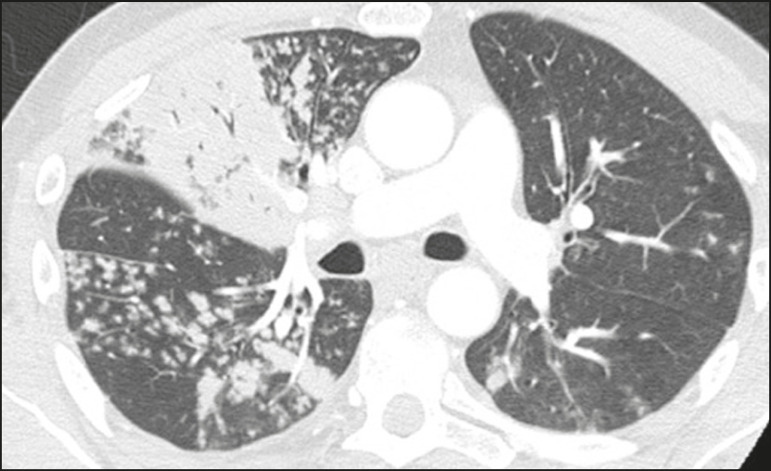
Atypical viral pneumonia pattern on high-resolution CT of the chest, with multiple centrilobular pulmonary nodules accompanied by parenchymal consolidation with air bronchograms. The patient had a confirmed diagnosis of pulmonary tuberculosis.Negative examination for viral pneumonia-examination without significant pulmonary changes or with changes unrelated to infection
